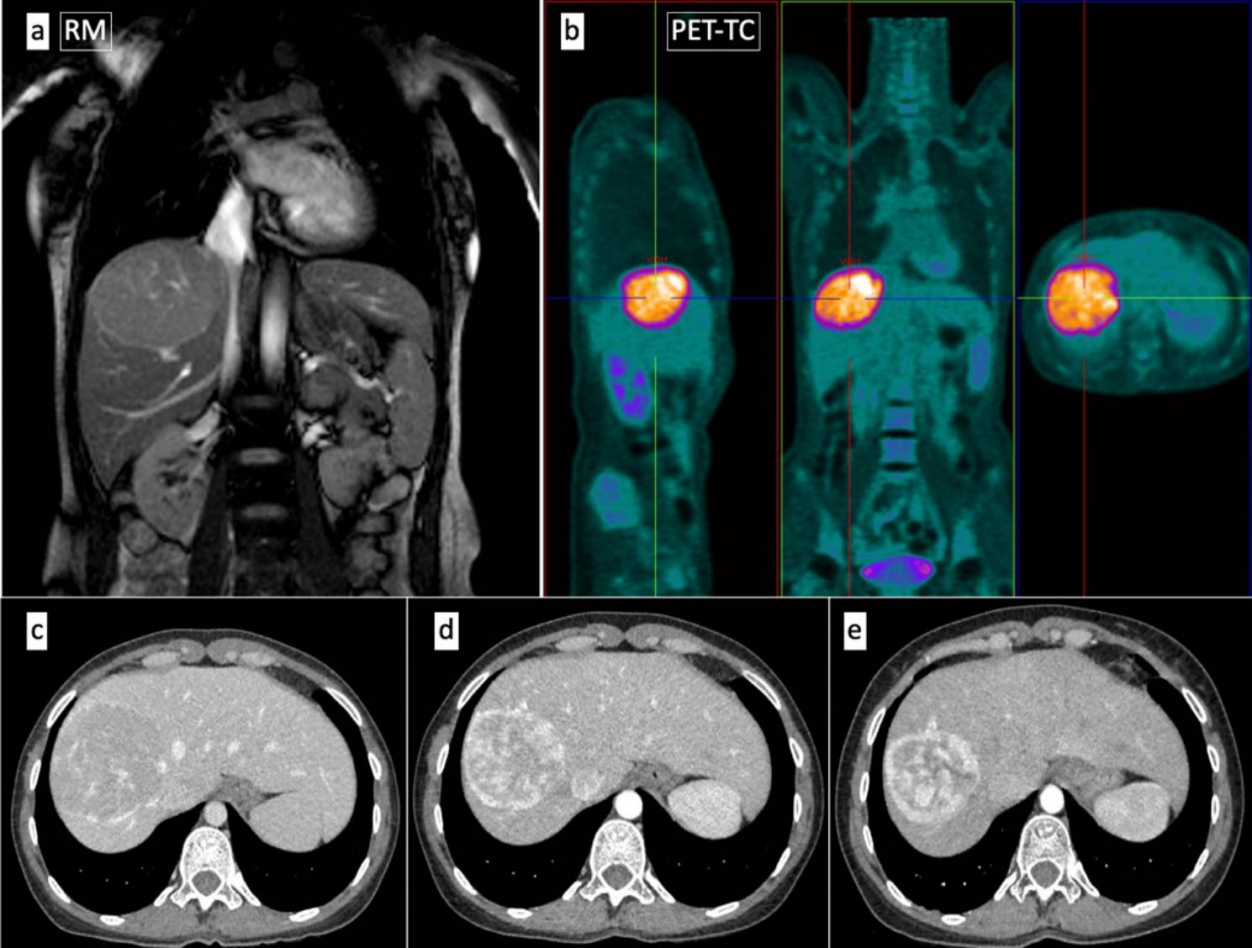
MRI image of a solid mass involving segment VII of the right hepatic lobe (a). Hypermetabolic, hypodense, and homogeneous hepatic lesion (SUV max = 12,6) of demarcated boundaries (b). Comparative study of contrast-enhanced CT-scan every two months with a gradual reduction in size over time (c, d, and e)
